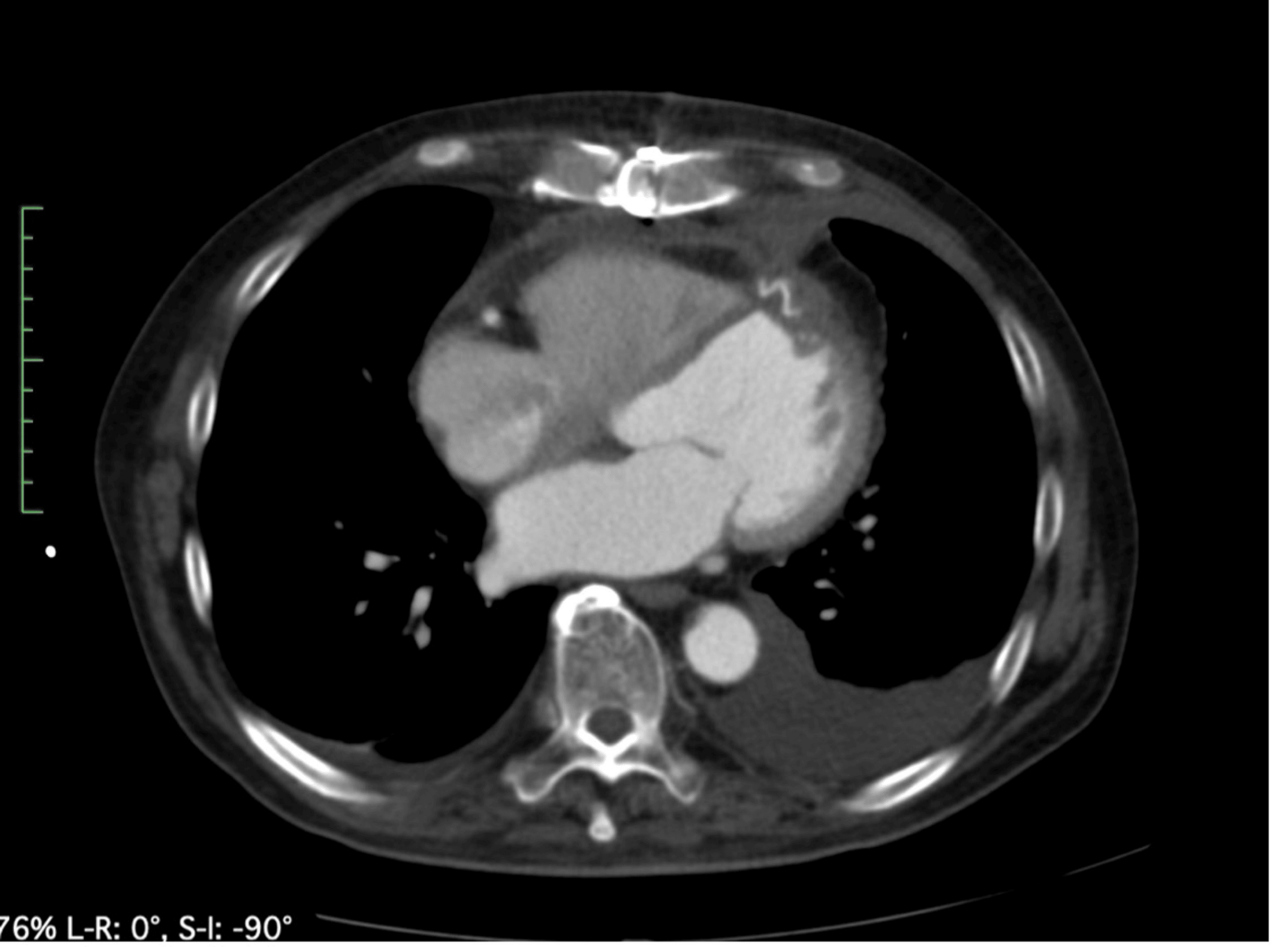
Computed tomography (seventh postoperative day) confirming the repair of the pseudoaneurysm
